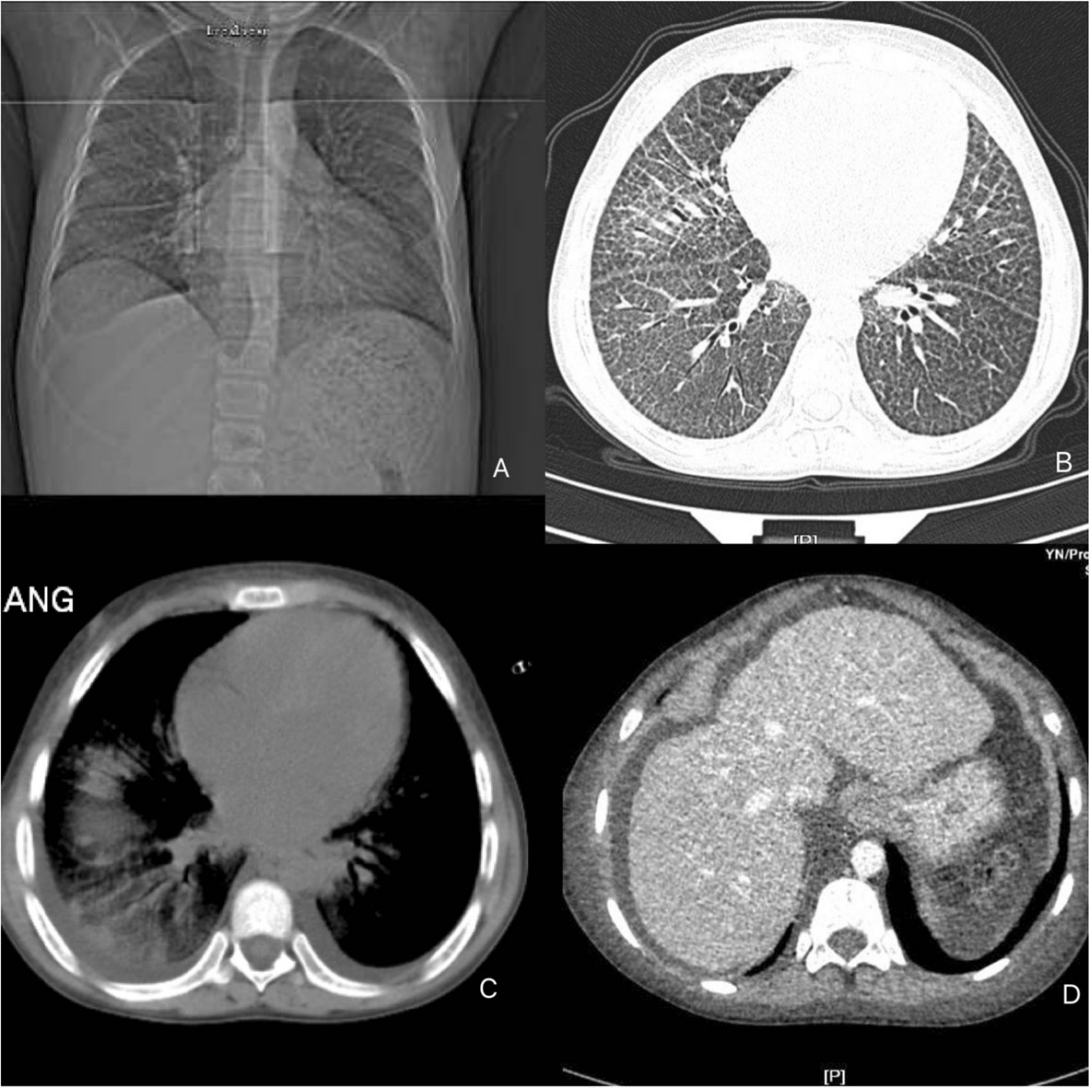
a Anteroposterior chest X-ray showing a bilateral reticulonodular interstitial pattern. b Computed tomography pulmonary window showing bilateral reticular change, ground-glass opacity of the lung parenchyma, characteristic of the paving pattern, and pulmonary interstitial fibrosis. c Computed tomography mediastinal window showing exudation, consolidation, and a small amount of pleural effusion. d Enlarged liver with irregular shape
